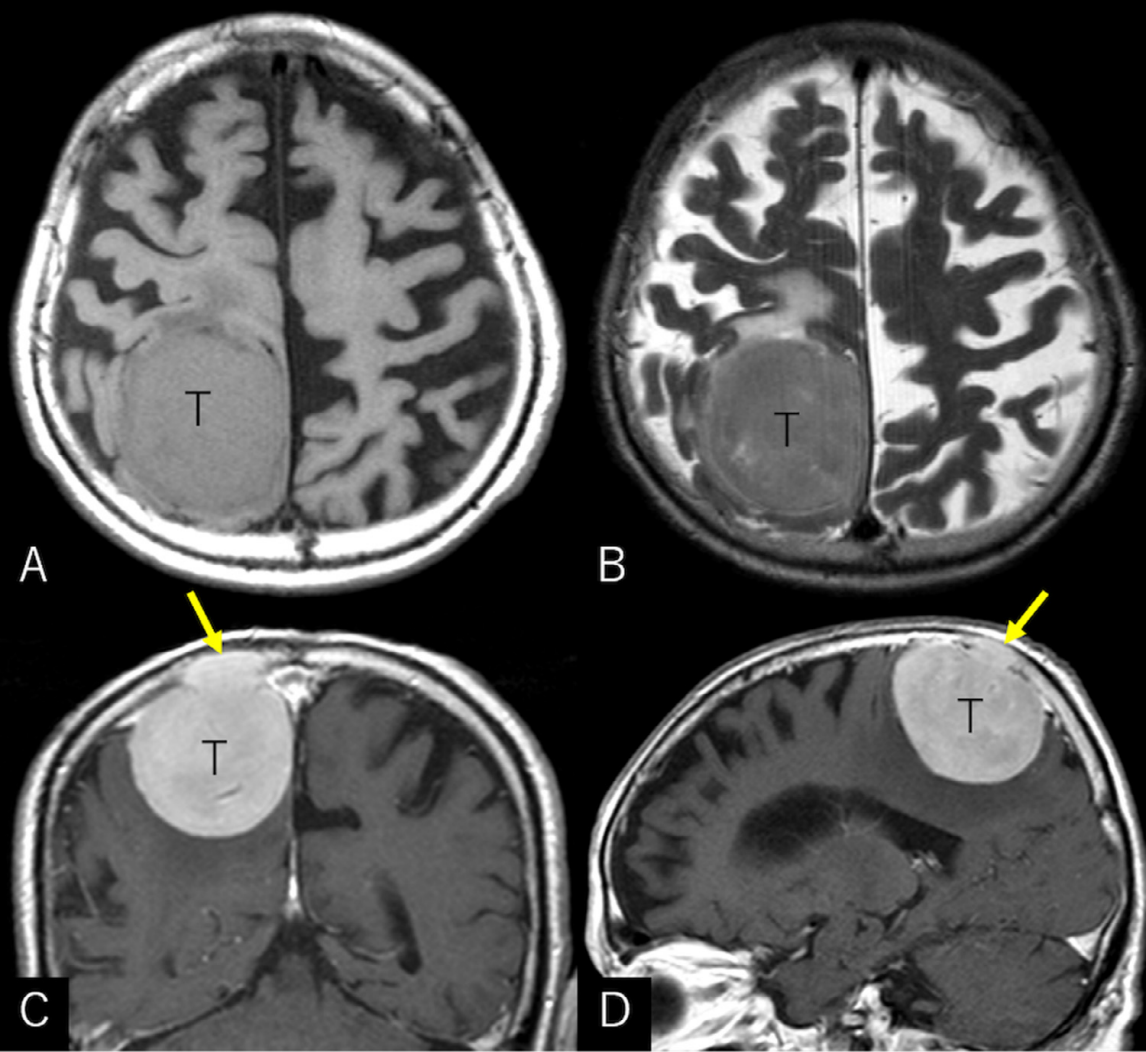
Axial T1- (*A*), T2-weighted (*B*), and postcontrast coronal (*C*) and sagittal (*D*) MRIs showing an intensely enhancing extra-axial mass. It appears isointense on T1- and hyperintense on T2-weighted sequences, respectively, broad-based on the convexity dura mater, and measuring 52 × 46 × 48 mm in maximal dimensions, with a portion upwardly protruding into the eroded skull.
